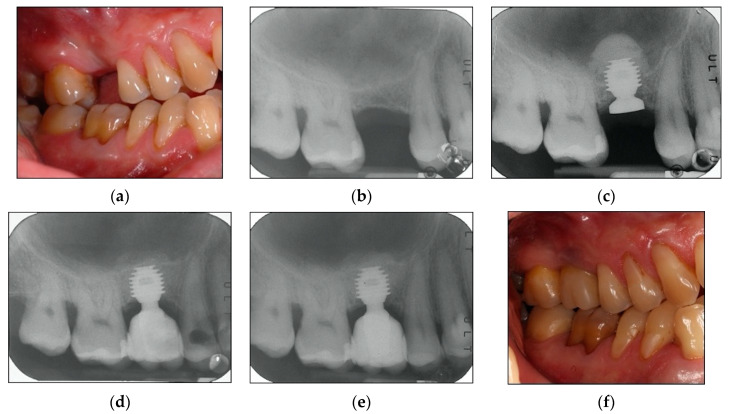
(a–f). Clinical case: Single implant placed in 1.6 site. (a) Clinical photograph before implant placement, missing first molar. (b) Pre-operative radiograph before implant placement in site 1.6. See minimal bone in implant site. (c) Radiograph obtained at implant placement. See short implant with sinus lift temporary abutment designed to prevent displacement of the implant into the sinus, also see augmented sinus floor. (d) Radiograph obtained at time of loading. See augmented sinus floor, also see the radiolucent area in the crown of the second bicuspid, which was a lost restoration. (e) Radiograph obtained at three-year follow-up. See stable bone levels, also see the replaced restoration in the crown of the second bicuspid. (f) Clinical photograph at three-year follow-up. See stable clinical conditions.
